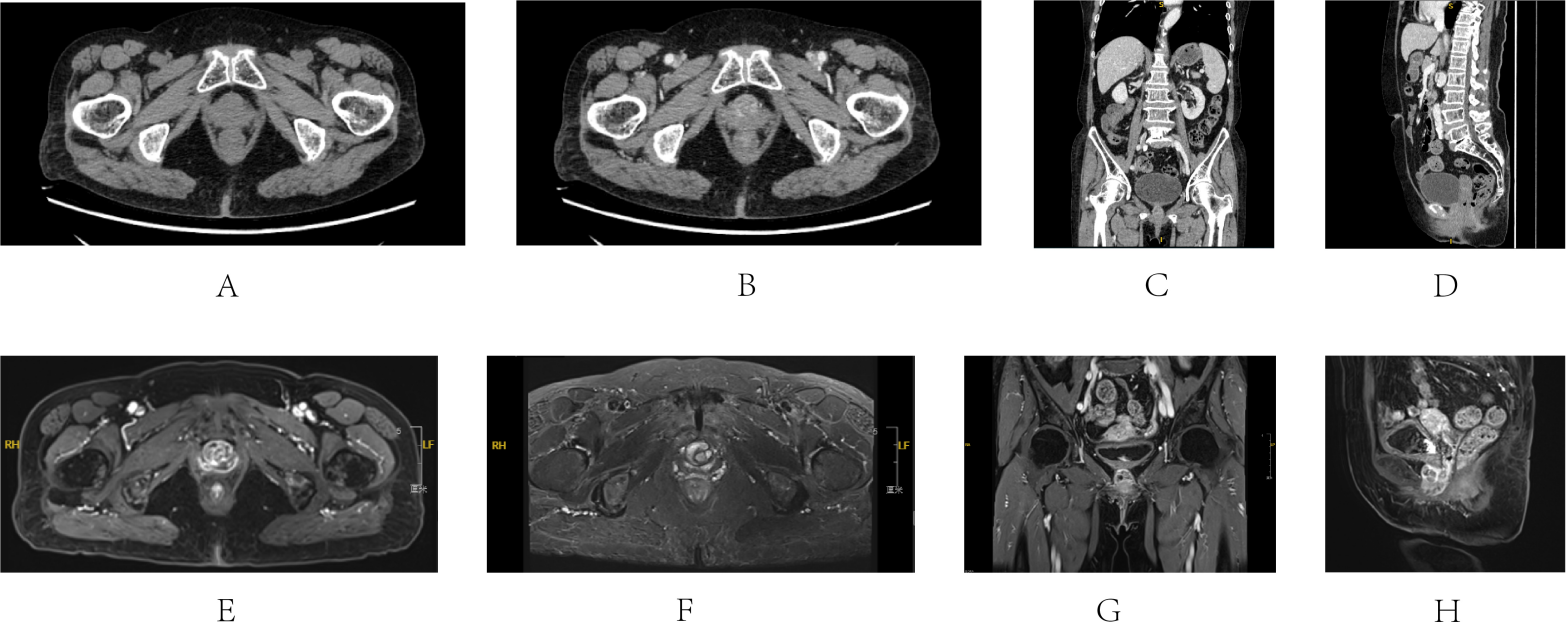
CT and MRI images of Case 1’s first visit to the hospital (March 2022). CT (A-D) A 2.5*2 cm nodule was found on the urethra, with a plain CT value of 45 HU, and enhancement was visible. MRI (E-H) A 2.7*2.2 cm cystic signal was seen with long signal at both T1 and T2 stage, and significant enhancement of the margins and cystic septa can be seen. (A) Axis plain CT scan of the mass; (B) Axis enhanced CT scan; (C) Coronal enhanced CT scan; (D) Sagittal enhanced CT scan; (E) Axis T1 stage of enhanced MRI; (F) Axis T2 stage of enhanced MRI; (G) Coronal T1 stage of enhanced MRI; (H) Sagittal T1 stage of enhanced MRI.
